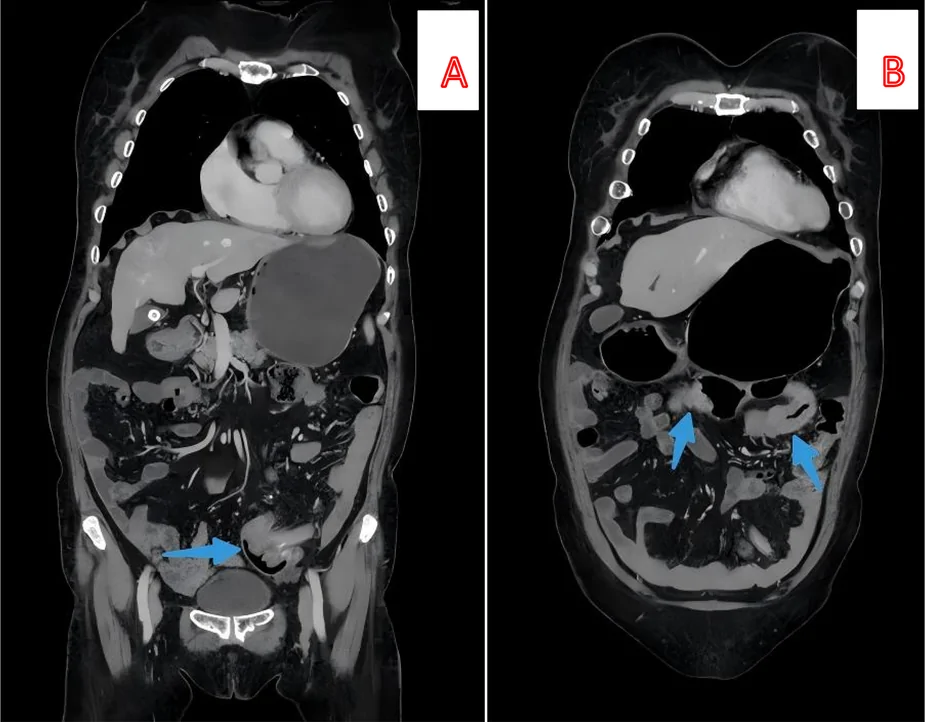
Preoperative enhanced CT images of sigmoid colon tumor (A) and transverse colon tumor (B) the area indicated by the blue arrow is the thickened part of the colon wall that is considered to be a tumor.
